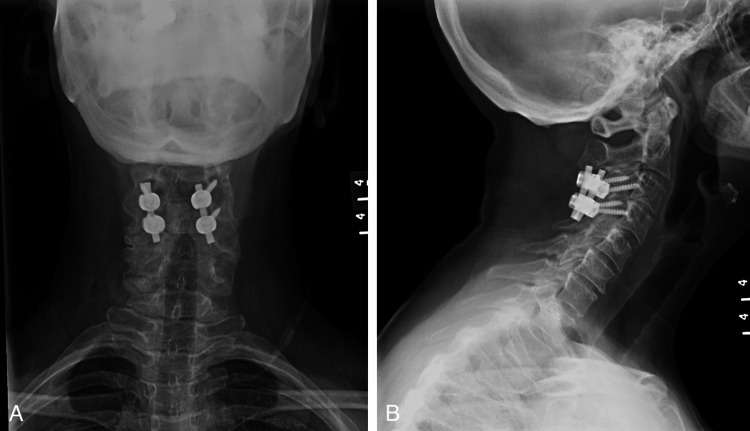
(A, B) Post-operative radiographs obtained at the one-year post-operative visit demonstrating reduced C3-4 facets with C3-4 posterior instrumentation.
